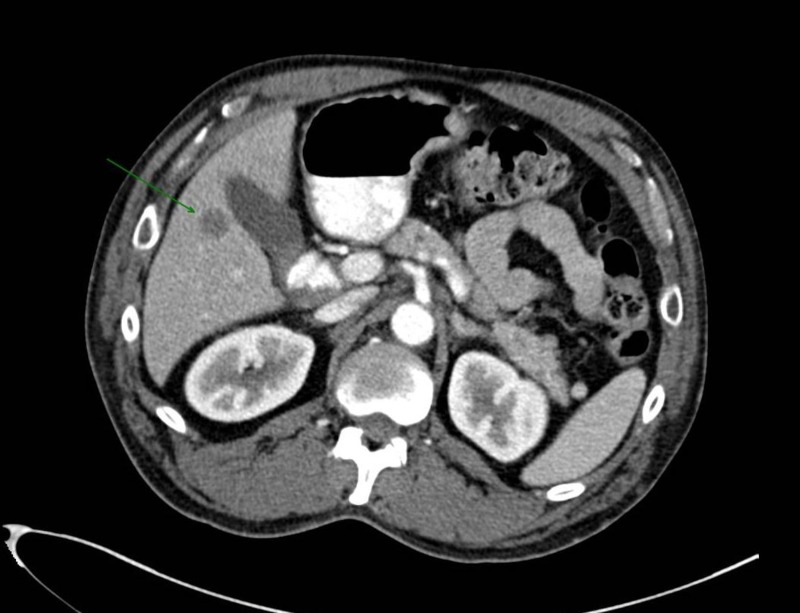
Computed tomography (CT) scan of the abdomen. The arrow indicates a 4 mm hypoattenuating lesion in the liver, which along with the other smaller ones, reflects metastatic disease in the setting of a primary squamous cell carcinoma of the lung.
